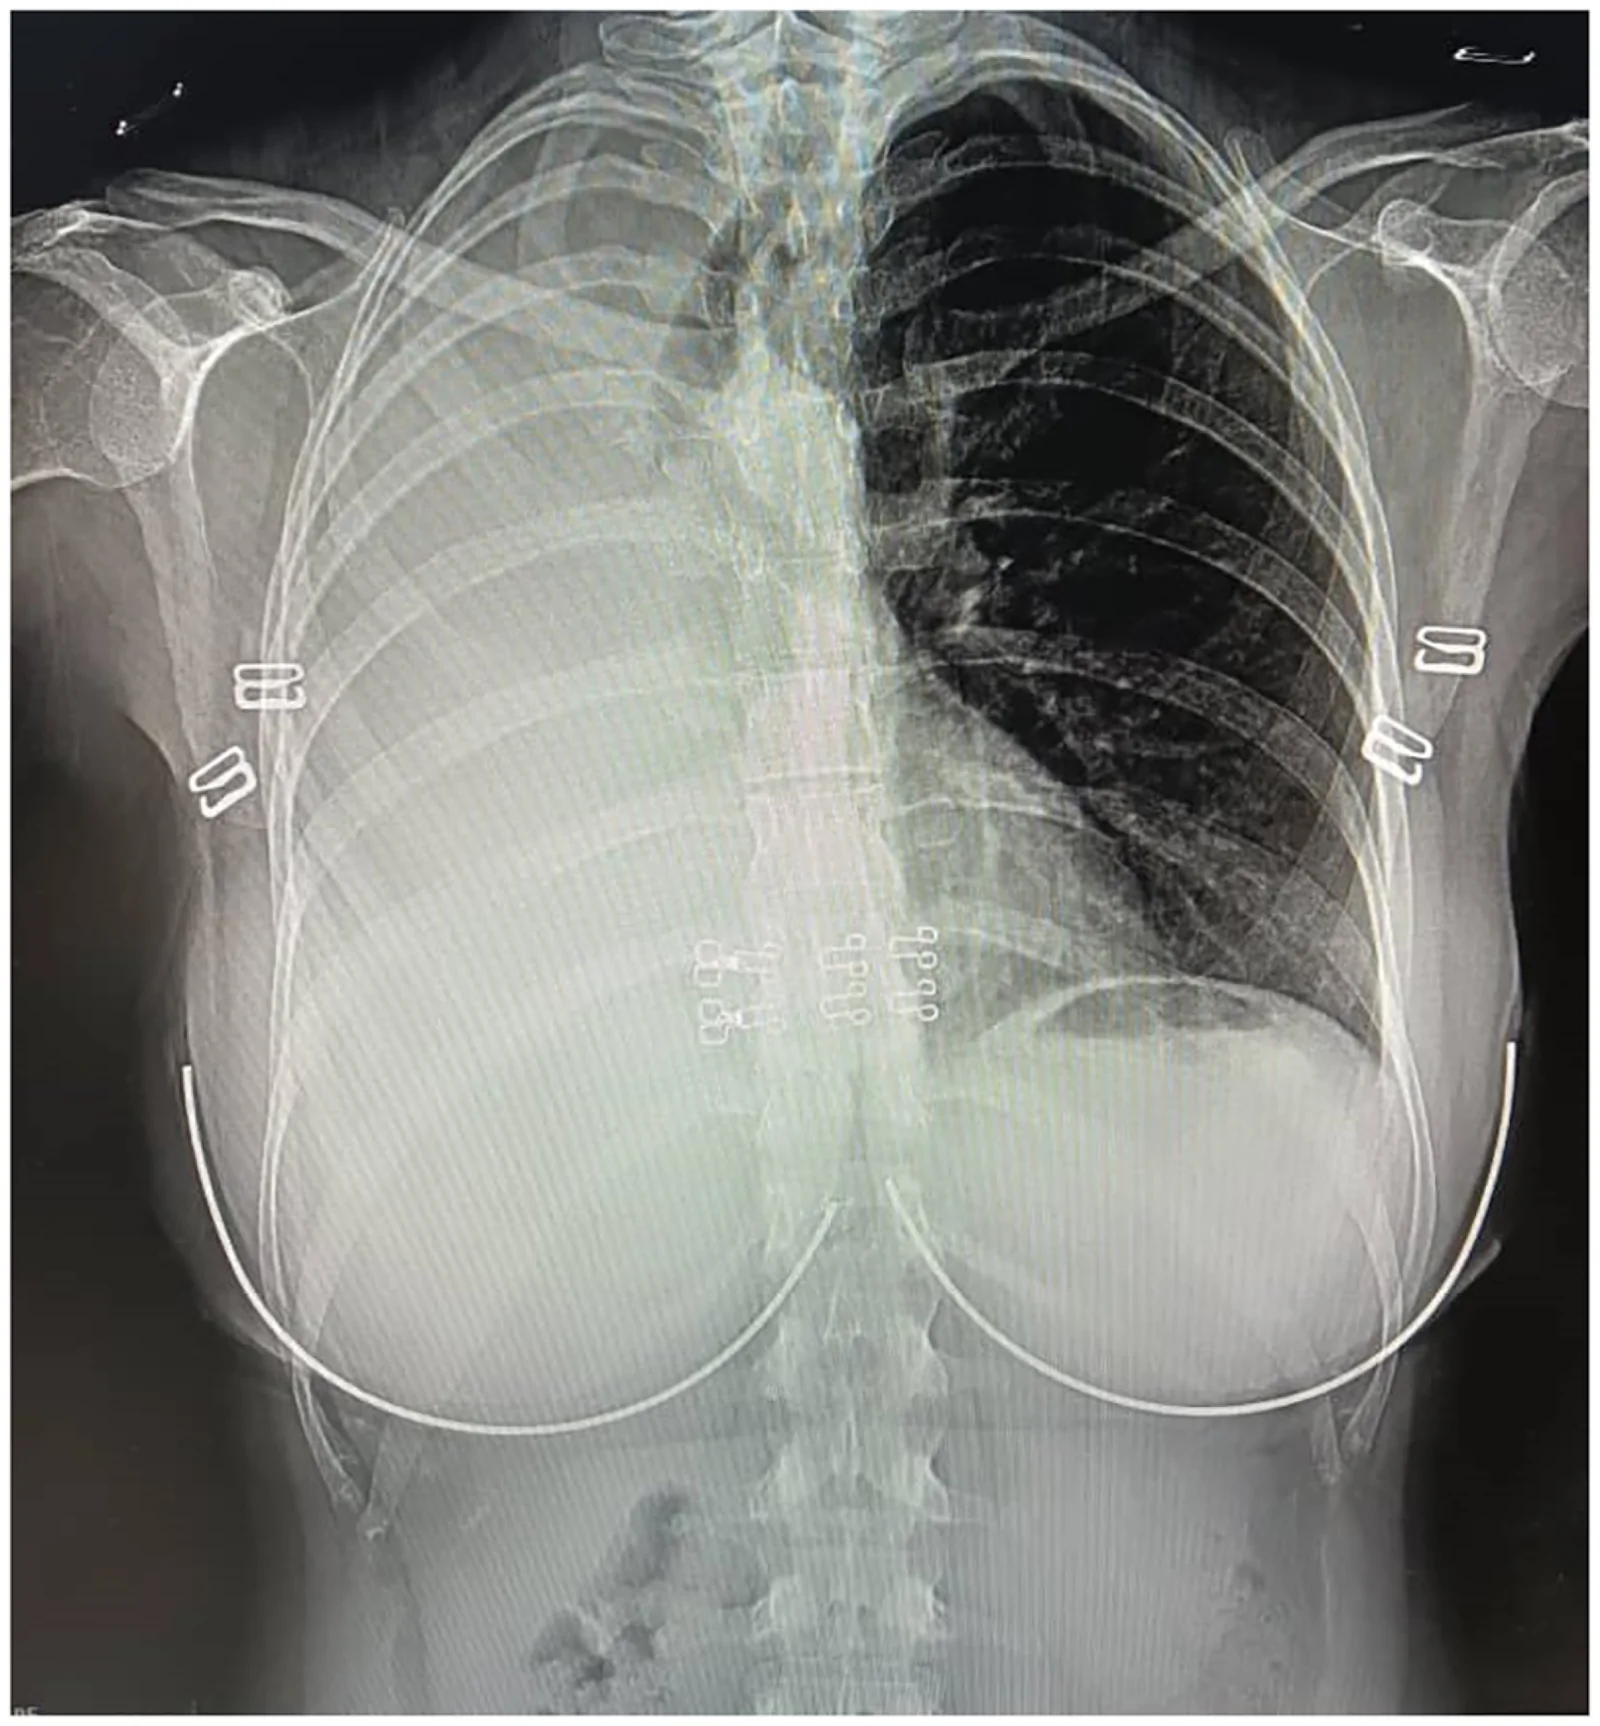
Preoperative chest radiograph. Chest radiograph demonstrating complete opacification of the right hemithorax with ipsilateral tracheal and mediastinal deviation, consistent with total right-lung atelectasis and marked volume loss.
